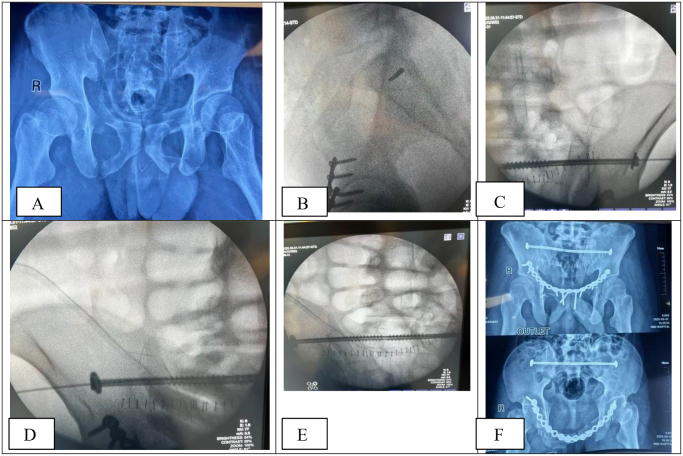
(A) AP pelvic radiograph showing bilateral superior and inferior pubic rami fractures with SIJ disruptions. (B) Lateral fluoroscopic view showing the left-sided guidewire entry point for an S1 ilio-sacral screw. (C, D) A 6.5 mm partially threaded cannulated screw was placed first on the left, then on the right, using the same guidewire. (E) Outlet fluoroscopic image post-fixation showing bilateral ilio-sacral screws placed over a single guidewire. (F) Inlet and outlet view post-fixation demonstrating a well-stabilized pelvic ring with anterior plating and bilateral posterior sacroiliac screws.
